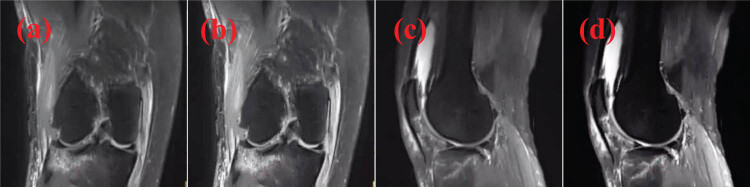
Analysis of MRI images of a male patient aged 37 years with proximal tibia fracture (a and c: original images; b and d: SRR MRI images).
